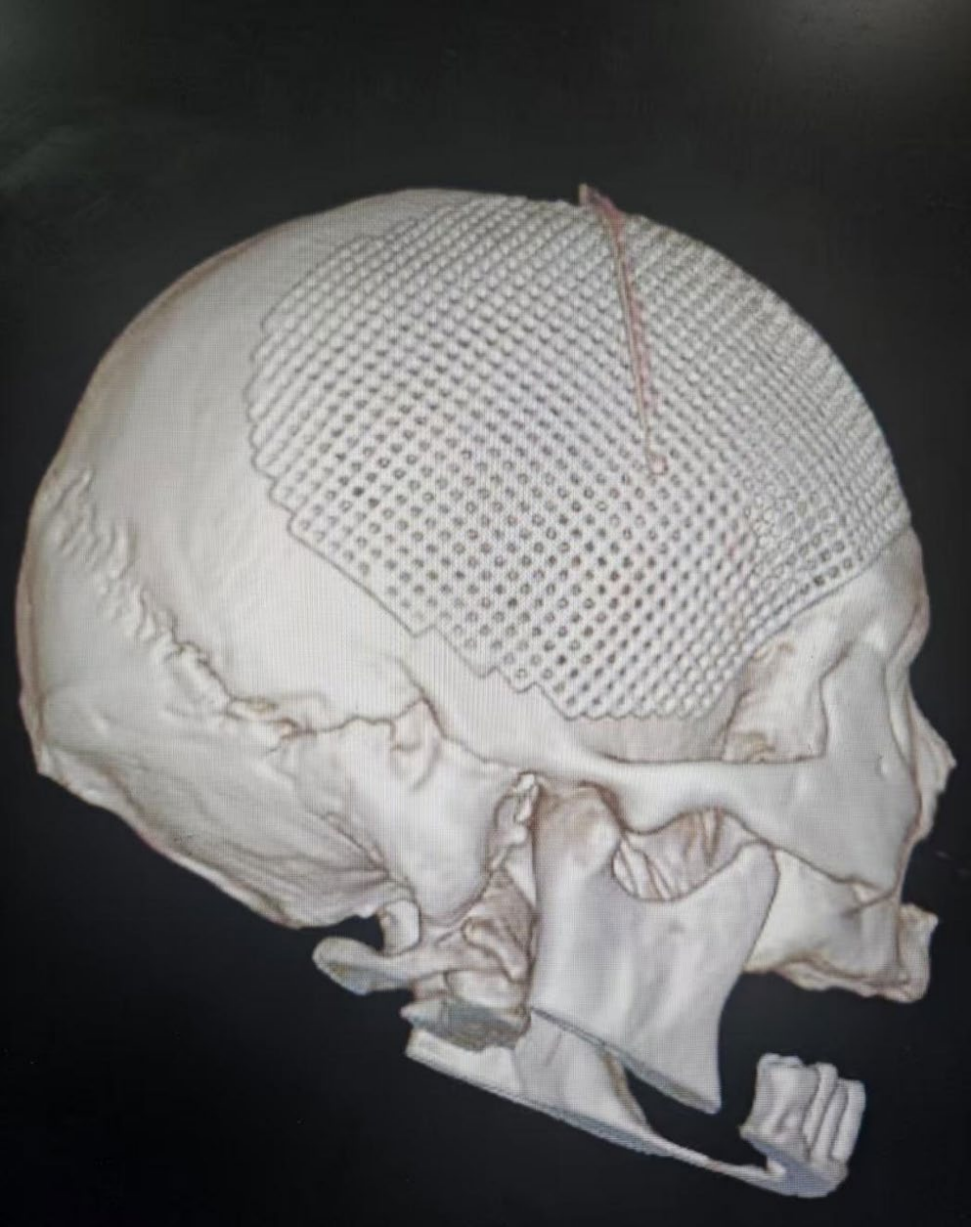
Cranial CT was re-examined within 24 h postoperatively (Reconstructed image)
